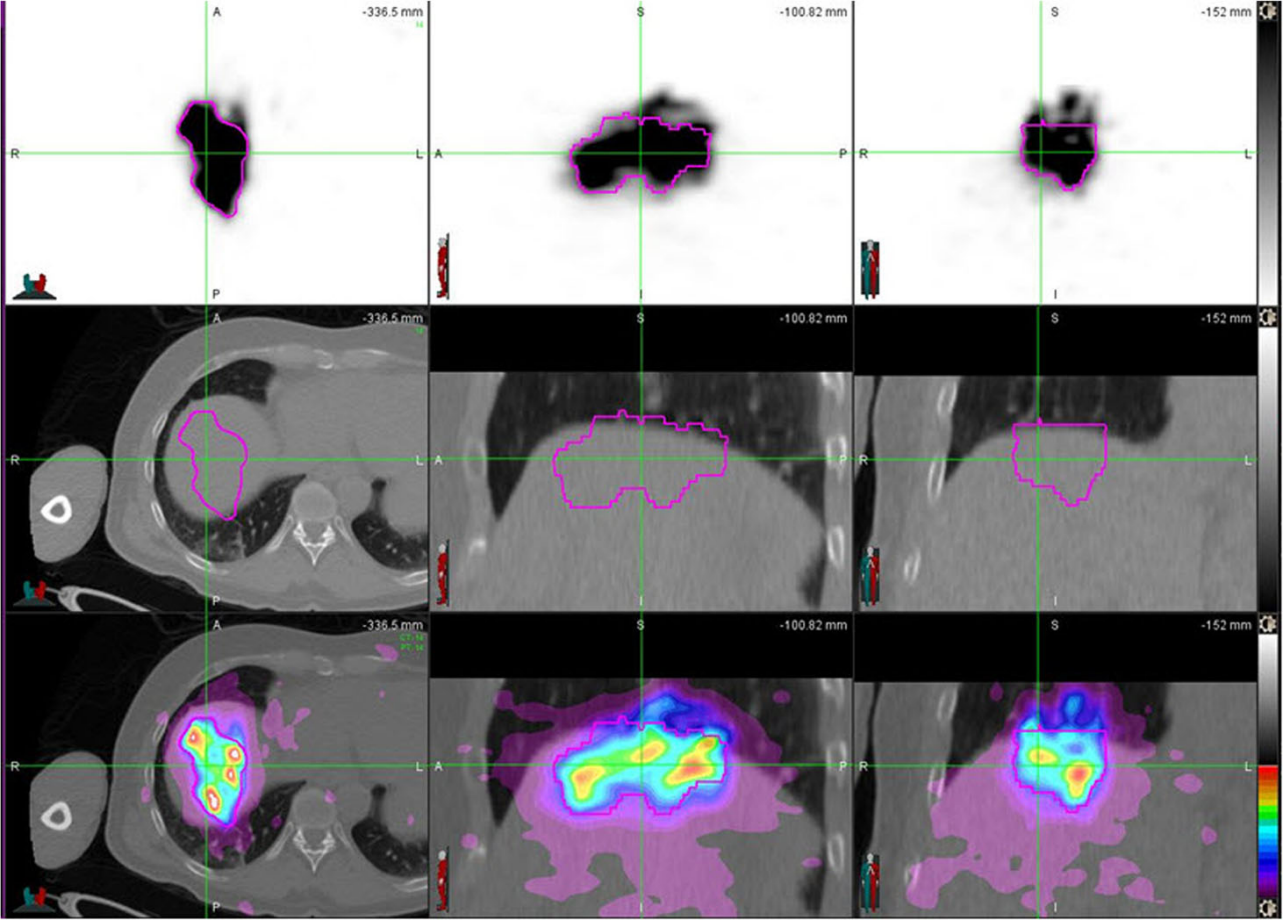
First row shows PET ^90^Y images, second row corresponding CT images, and third row fused PET/CT images. In fused coronal and sagittal PET/CT images, one can clearly see ^90^Y spillover into lungs due to respiratory motion artifacts
